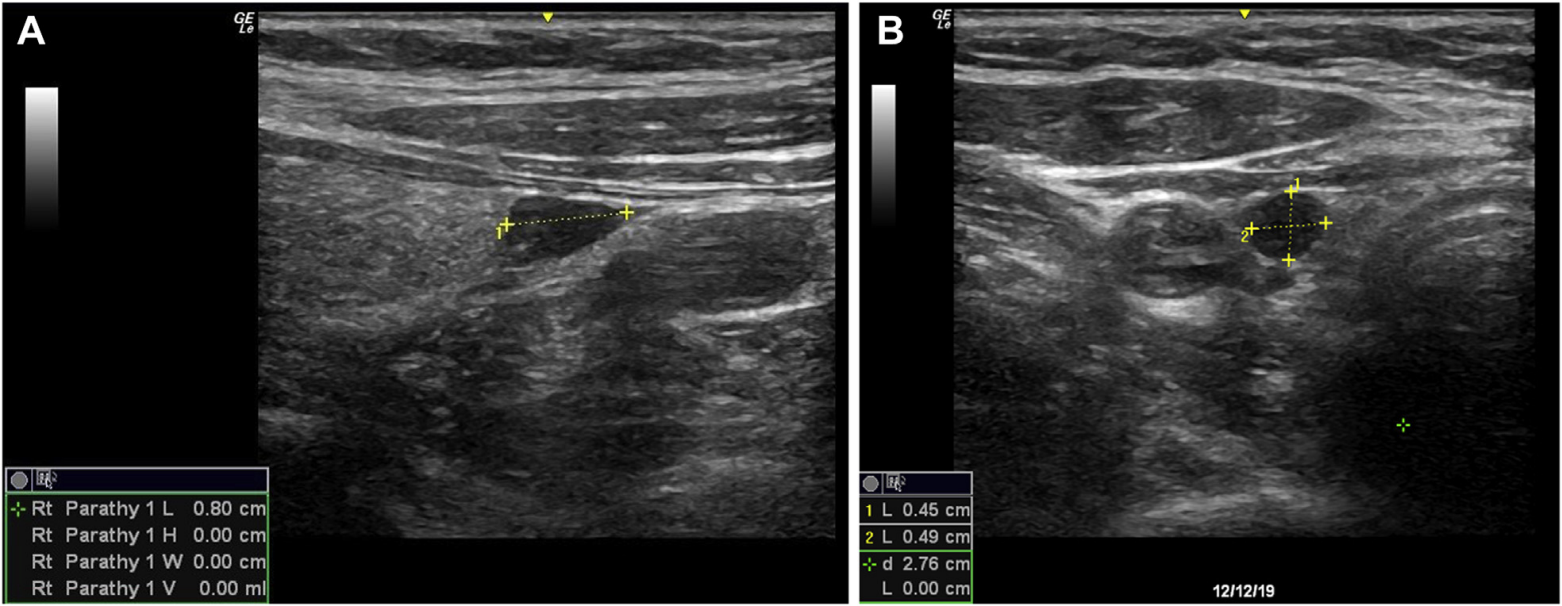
Ultrasound imaging of the parathyroid adenoma. The patient’s ultrasound scan revealed a 6-mm adenoma of the lower portion of the right parathyroid gland. *A*, Sagittal orientation. *B*, Transverse orientation.
